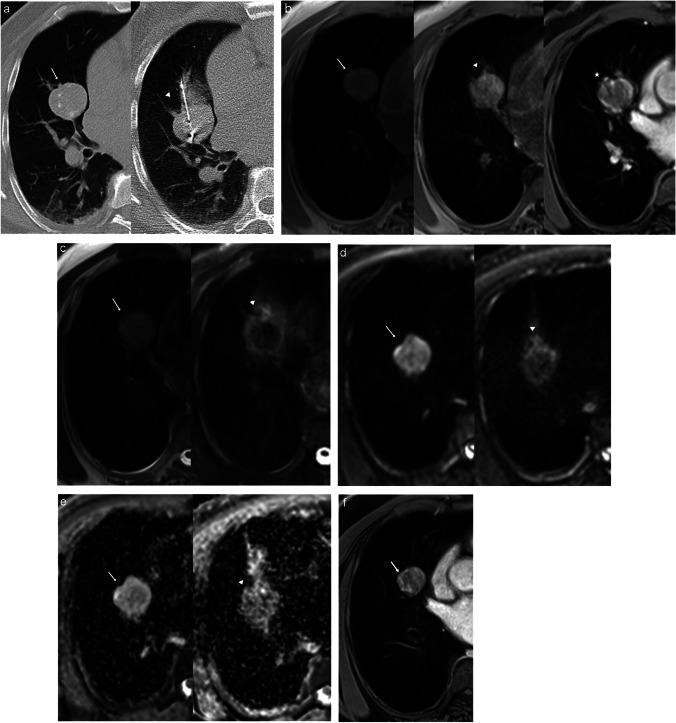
A 50-year-old male patient with renal cell carcinoma. The patient developed metachronous right pulmonary metastasis. a Combined CT images showing the pre-ablation metastatic lesion (left-image arrow) and during MWA (arrowhead). b Combined MRI images in T1WI pre-ablation (left image), T1WI 24 h post-ablation (middle image), and T1WI with contrast 24 h post-ablation (right image) displaying low signal intensity before and after ablation with irregular thick marginal contrast enhancement after ablation. c Combined MRI images in T2WI pre-ablation (left image) and T2WI 24 h post-ablation (right image) displaying high signal before ablation and central low signal intensity with marginal high signal after ablation. d DWI pre-ablation (left image) and DWI 24 h post-ablation (right image) displaying high signal intensity before ablation with reduction of the high signal intensity after ablation. e ADC map pre-ablation (left image) and ADC map 24 h post-ablation (right image). f T1WI post-contrast follow-up after 6 months showing nodular contrast enhancement of the lesion denoting residual local tumor activity
